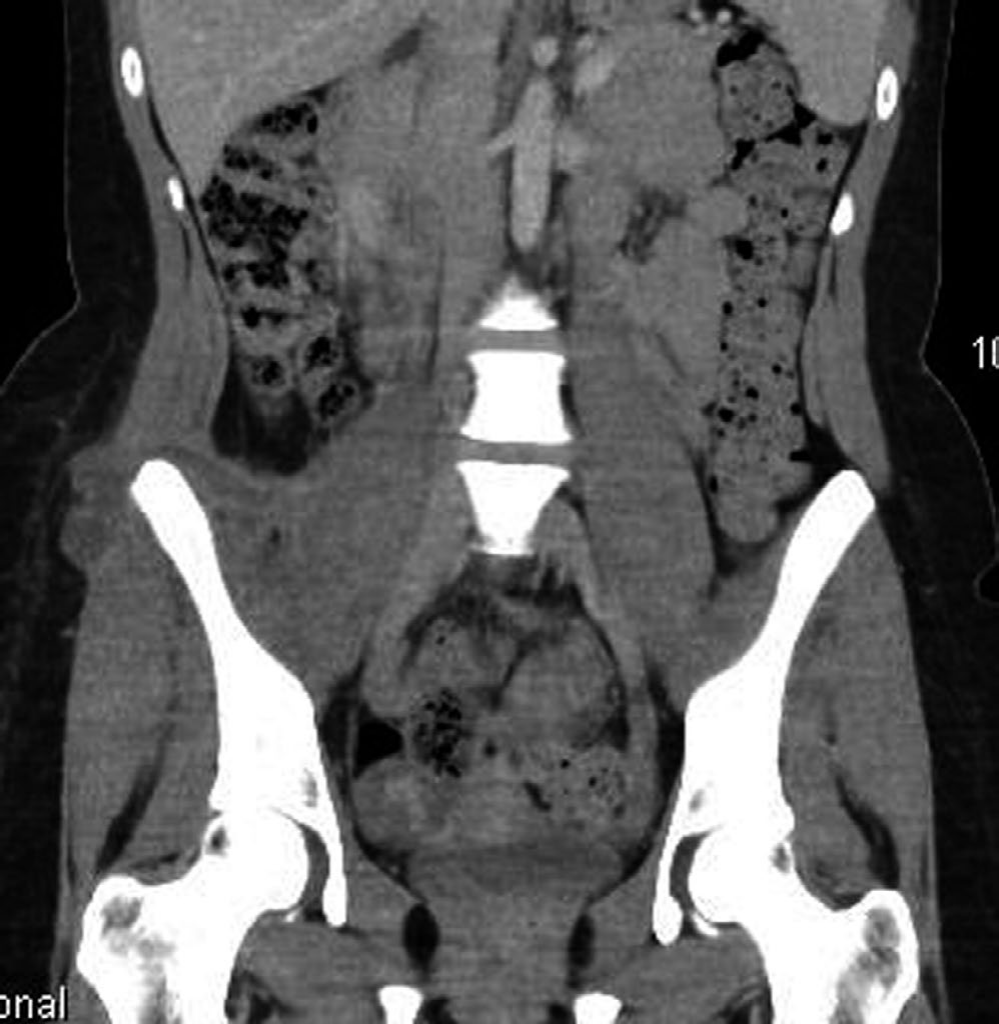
Computed tomography abdomen showing horseshoe shaped collection in right lateral abdominal wall into the subcutaneous fat
